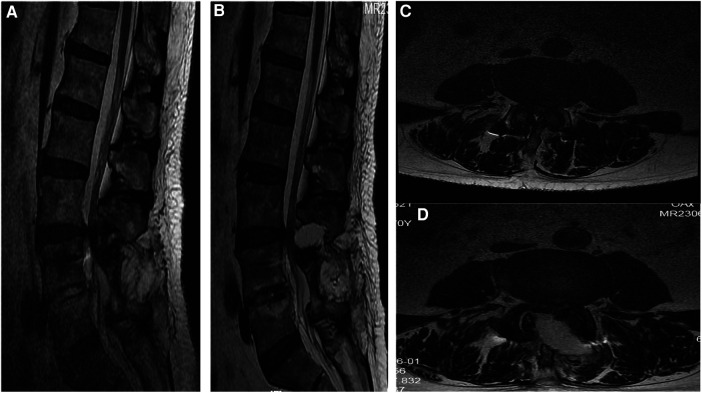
Preoperative and postoperative thoracic MRI. (A) Sagittal T2-weighted imaging showed spinal canal stenosis at the L3/4. (B) Three months after the operation, the sagittal view of the lumbar spine MRI showed satisfactory decompression at L3/4. (C) Axial t2-weighted imaging showing hypertrophy of the ligamentum flavum at the L3/4 level, causing spinal stenosis. (D) Three months after the operation, the lumbar spine MRI axial view showed satisfactory decompression at L3/4.
